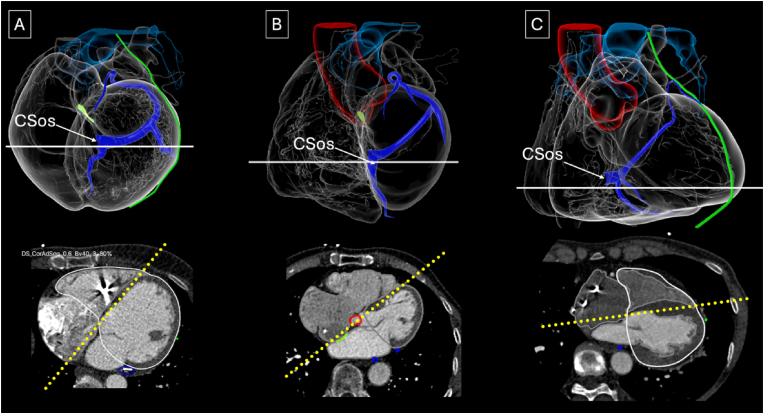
Impact of cardiac dilatation on coronary sinus ostium position: implications for fluoroscopic projections. (A–C) Upper panels: 3D CT reconstructions (inHeart) in LAO projection. The white line indicates the inferior border of the CS ostium (CSos). Lower panels: corresponding axial CT slices with yellow dotted lines indicating the cardiac long axis orientation. With progressive cardiac dilatation, two key changes occur: (1) the heart assumes a more horizontal orientation, as reflected by the increasingly horizontal cardiac long axis, and (2) the CSos displaces inferiorly relative to the cardiac silhouette in LAO. This inferior displacement explains why standard fluoroscopic angles may be insufficient in patients with dilated cardiomyopathy—the CSos appears lower within the cardiac shadow than anticipated, requiring adjustment of both C-arm angulation and catheter approach. The green line indicates the left phrenic nerve course along the lateral pericardium. (For interpretation of the references to colour in this figure legend, the reader is referred to the Web version of this article.)
